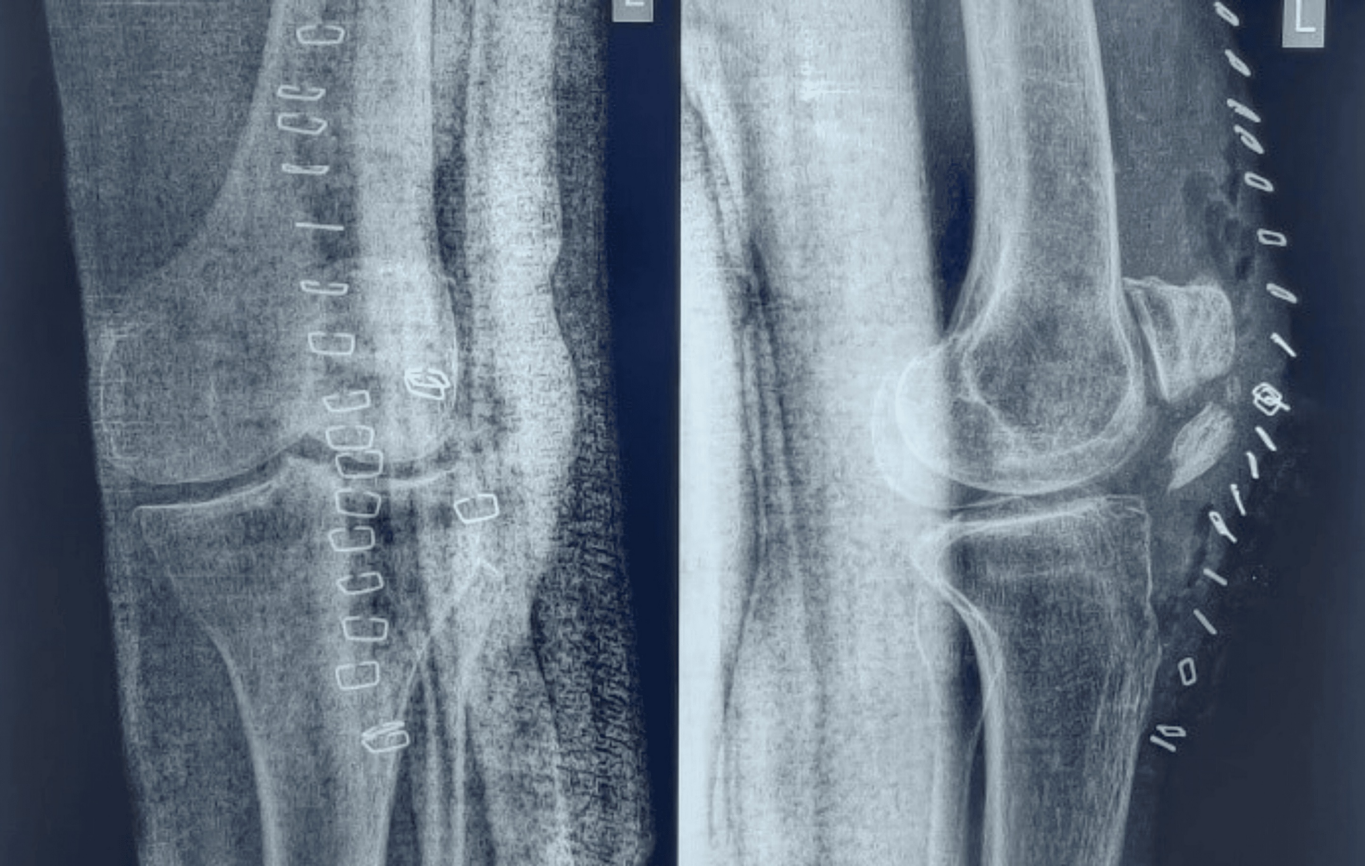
Immediate post-operative radiograph of the left knee joint after suturing the extensor mechanism by the Krackow technique
